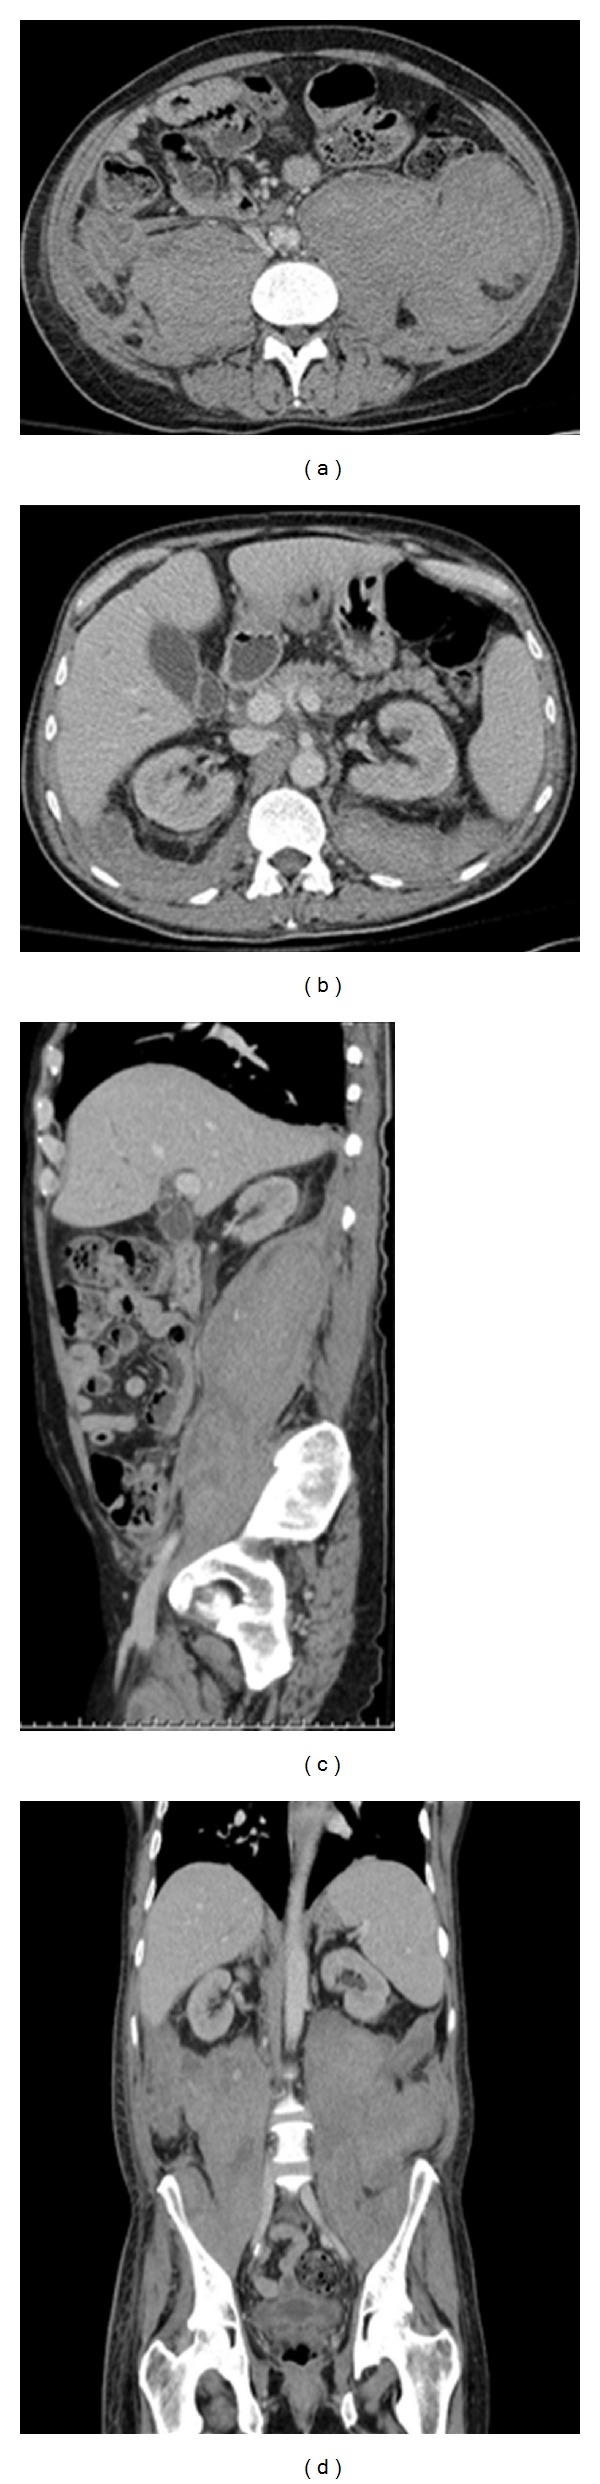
Repeat computed tomography of the abdomen and pelvis demonstrating hematoma stability. Better seen are mixed-aged bilateral retroperitoneal hematomas in the posterior pararenal spaces displacing both kidneys anteriorly with marked mass effect on peritoneal structures.
